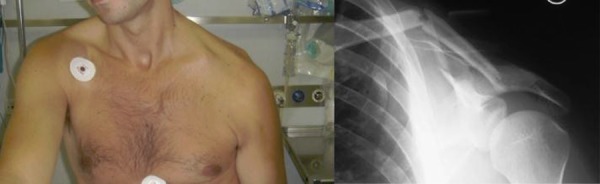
Clinical and Xray images of floating shoulder.
